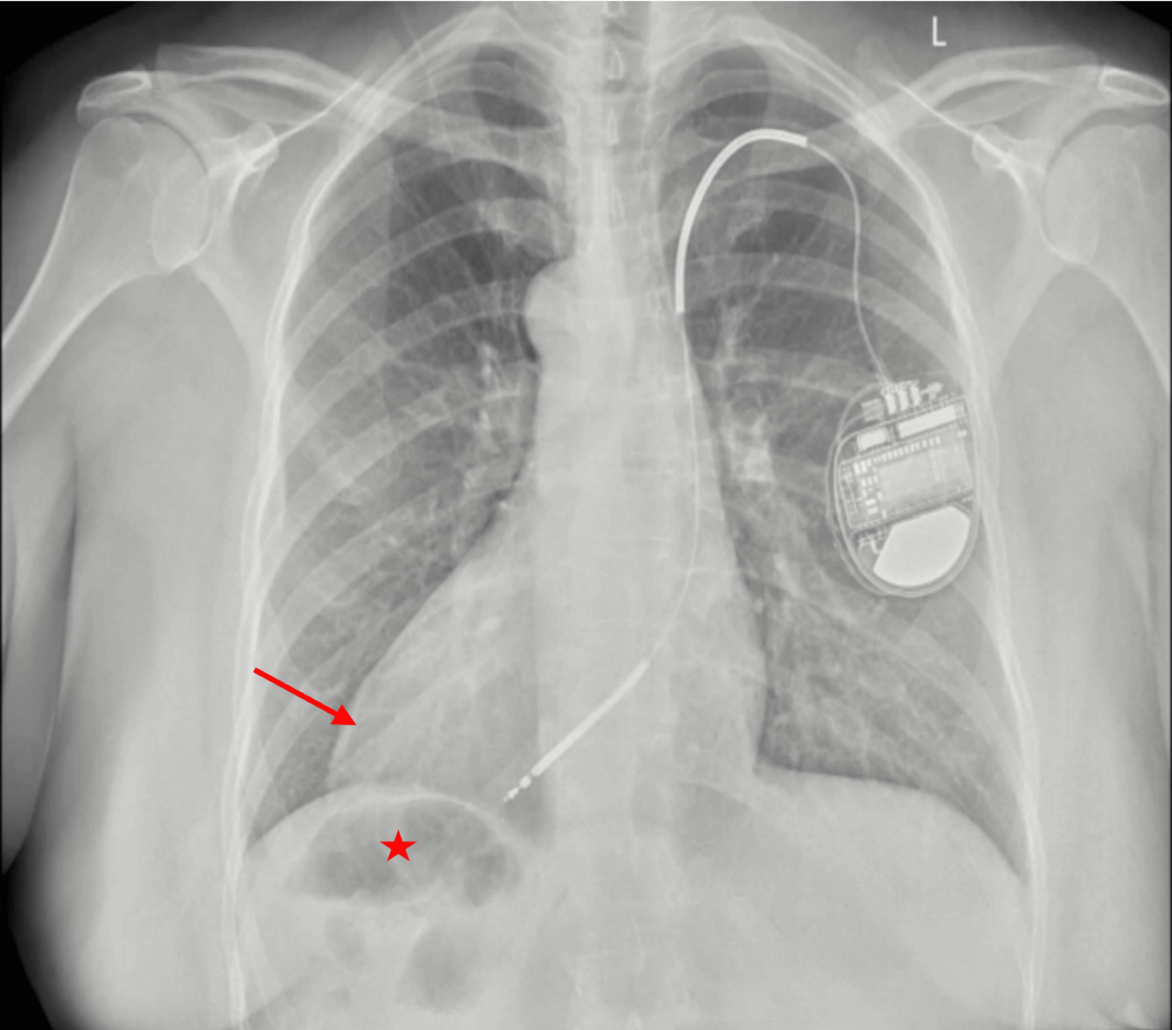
Chest X-ray The cardiac apex (arrow) is directed toward the right hemithorax, consistent with dextrocardia, and the stomach (star) is located in the right upper quadrant.
